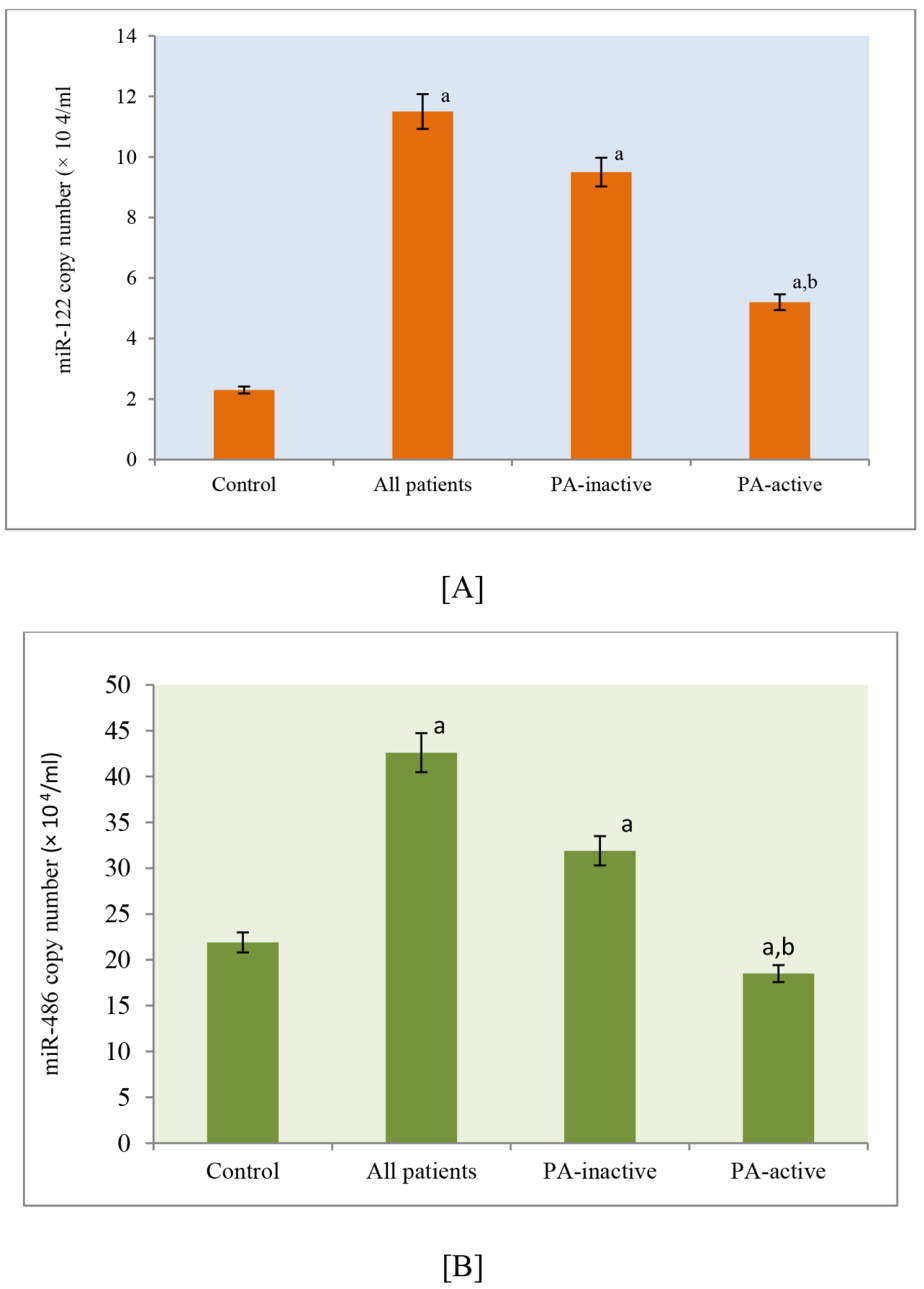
Elevation in serum miR-122 and miR-486 expression in both physically active and non-active chronic hepatitis C. Serum levels of miR-122 (A) and miR-486 (B) were compared between control subjects and CHC patients. The CT values of serum miRNA expression levels were transformed into absolute values using standard curves. The bars represent the levels corresponding to CT values ranging from 10 to 12 (2.9 × 104 − 0.75 × 104 copies/ml) for miR-122 and 40 to 45 (1.75 × 104 − 0.68 × 104 copies/ml) for miR-486, respectively
